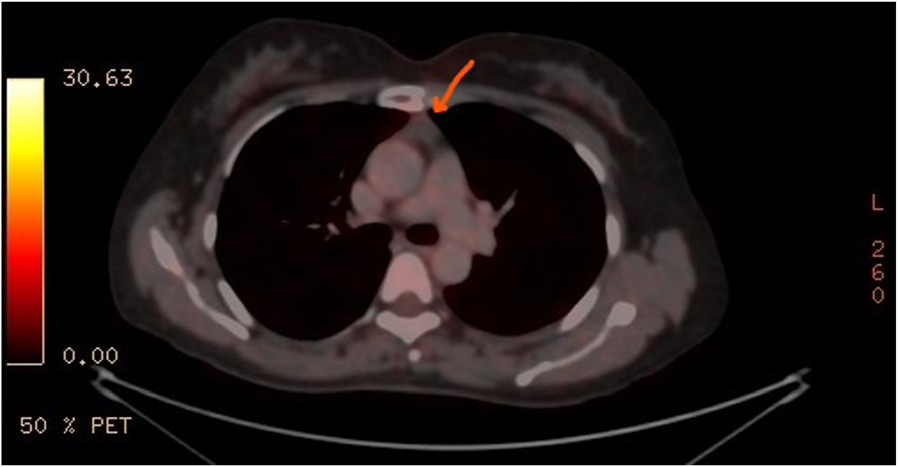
Integrated PET/CT demonstrating that the mediastinal lesion is not avid for FDG
